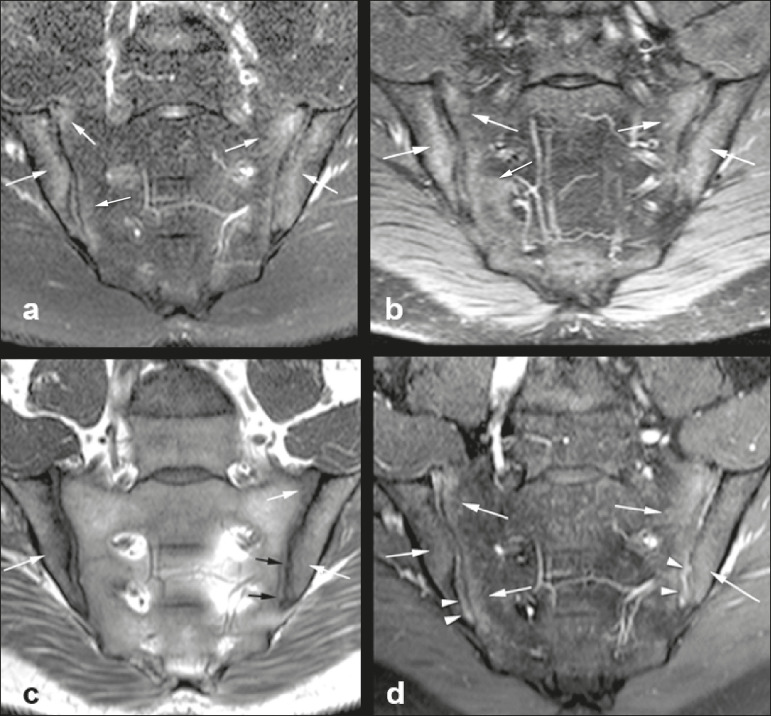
Male, 42 years old, diagnosed with ankylosing spondylitis. Coronal oblique MRI images. SPAIR T2 (a), STIR (b), T1 weighted without fat suppression (c), and SPIR T1 Gd + (d) sequences. The white arrows indicate areas of subchondral bone edema in both sacroiliac joints involving the iliac and sacral portions. The white arrowheads indicate intra-articular enhancement in the SPIR T1 Gd + sequence. The black arrows indicate areas of erosion on the iliac margin of the left sacroiliac.
